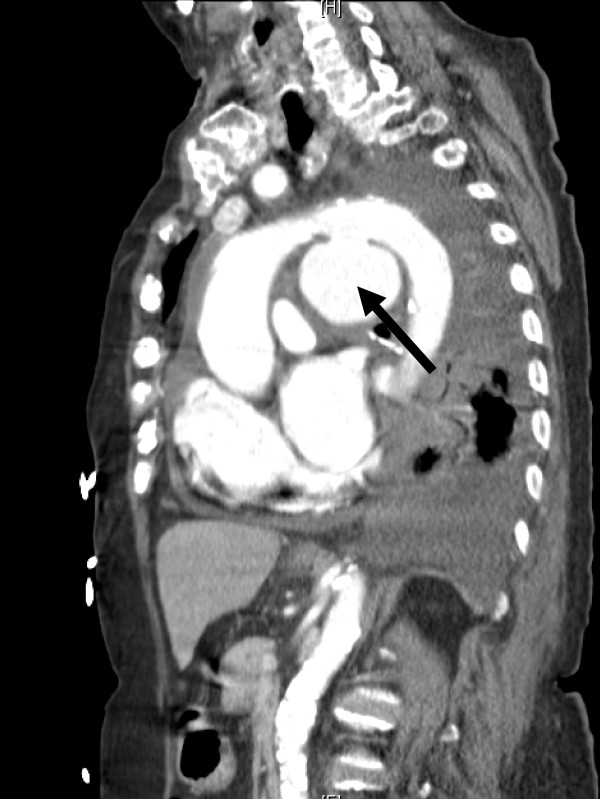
Chest computed tomography (CT) in sagital oblique reformation: a pseudoaneurysm size over 6*6 cm arises from aortic arch (black arrow) and suspicious hemorrhage into pericardium.
